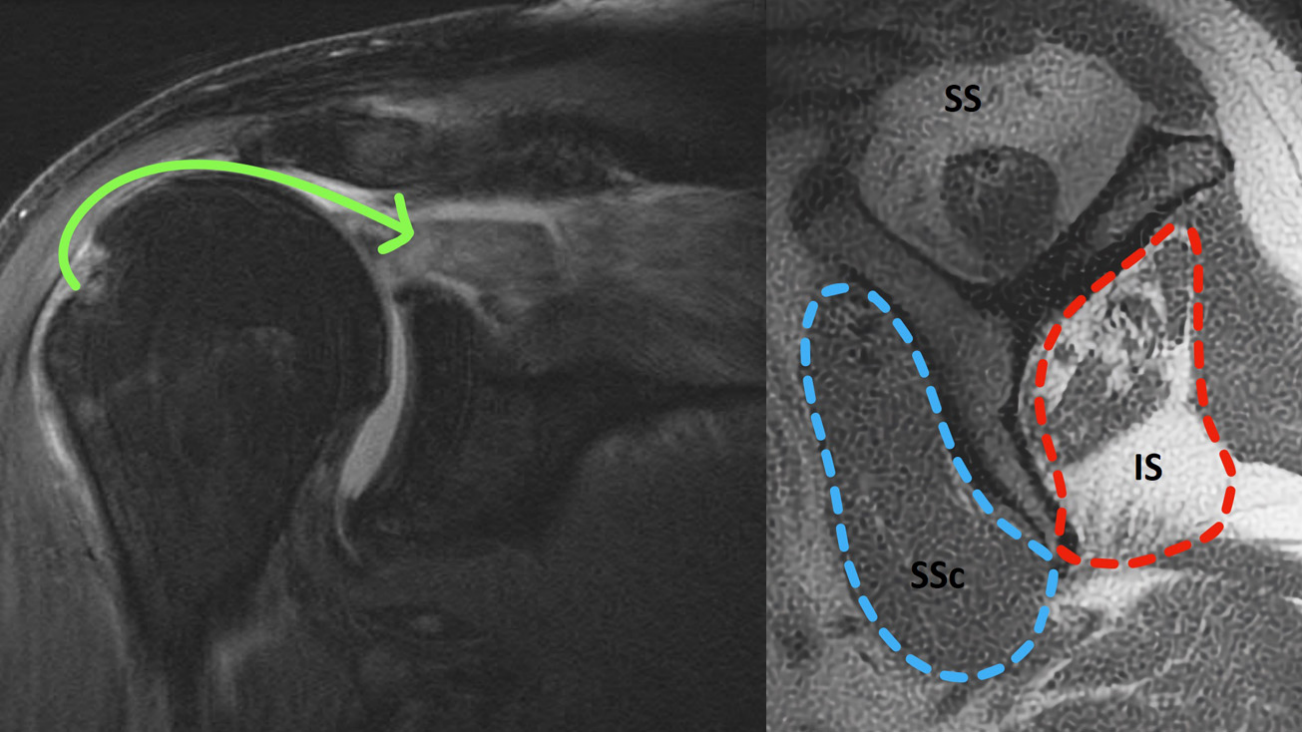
Preoperative coronal magnetic resonance imaging (MRI) demonstrating a retracted rotator cuff tear (arrow). Sagittal MRI demonstrating atrophy of the supraspinatus (SS) and infraspinatus (IS) muscles. SSc, subscapularis.
